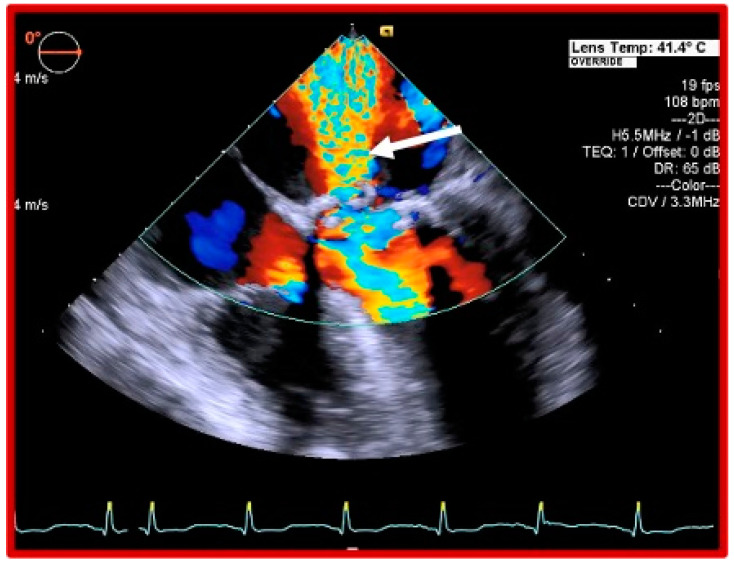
Doppler two-dimensional (2D) transesophageal ECHO showing a large mitral regurgitant jet.
